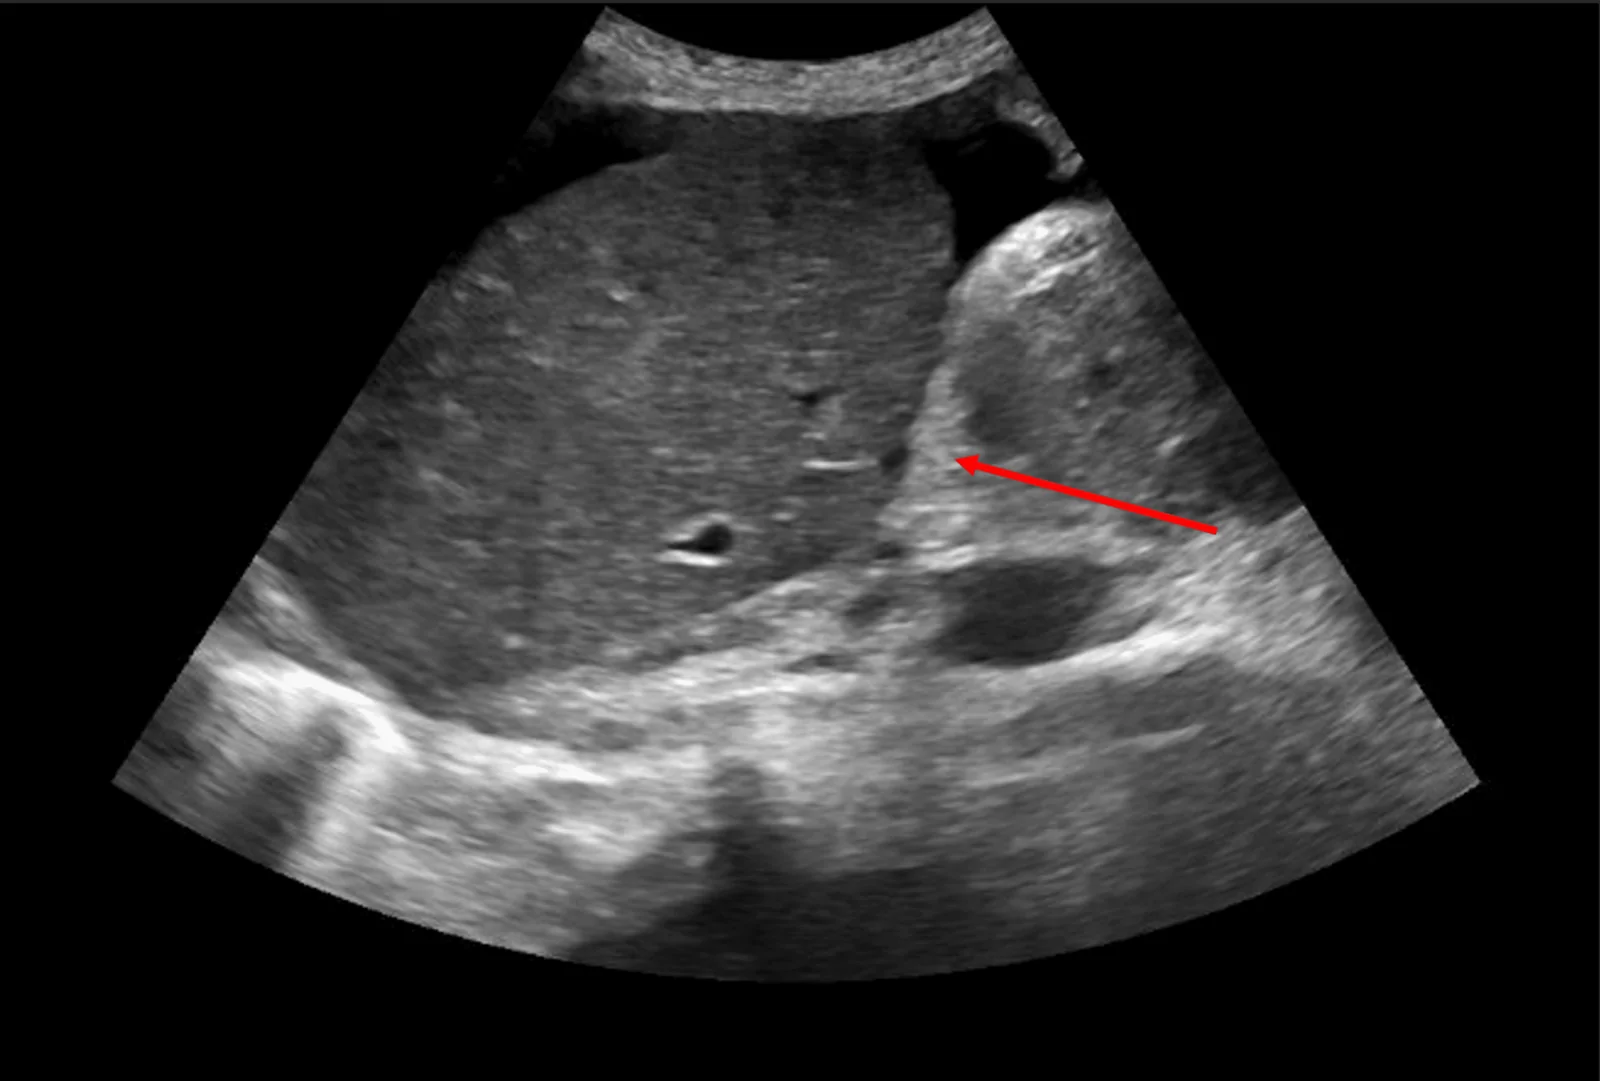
Right upper quadrant US demonstrating cirrhotic liver morphology Right upper quadrant US showing nodular hepatic contour and coarse parenchymal echotexture (arrow), findings concerning for cirrhosis. US: ultrasonography
